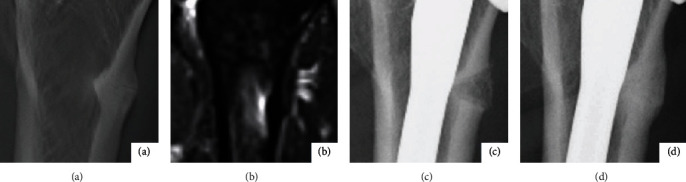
Anteroposterior radiograph showing a transverse thin fracture line with localized periosteal and endosteal thickening at the left subtrochanteric lateral cortex (a). Coronal STIR image showing focal cortical thickening with minimal edema of the adjacent bone marrow (b). Anteroposterior radiographs showing fracture region just after surgery (c) and complete bone repair at 7 months after surgery (d).
